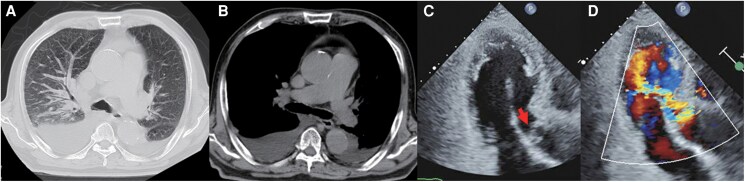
Computed tomography and transthoracic echocardiography findings. Chest computed tomography findings included pulmonary oedema, bilateral pleural effusion (*A*), and mild ascending aortic dilatation without evidence of aortic dissection (*B*). (*C*) Apical long-axis view on transthoracic echocardiography revealing aortic valve prolapse (arrow). (*D*) Colour Doppler imaging on transthoracic echocardiography demonstrating severe aortic regurgitation with an eccentric jet. CT, computed tomography; TTE, transthoracic echocardiography; AR, aortic regurgitation.
